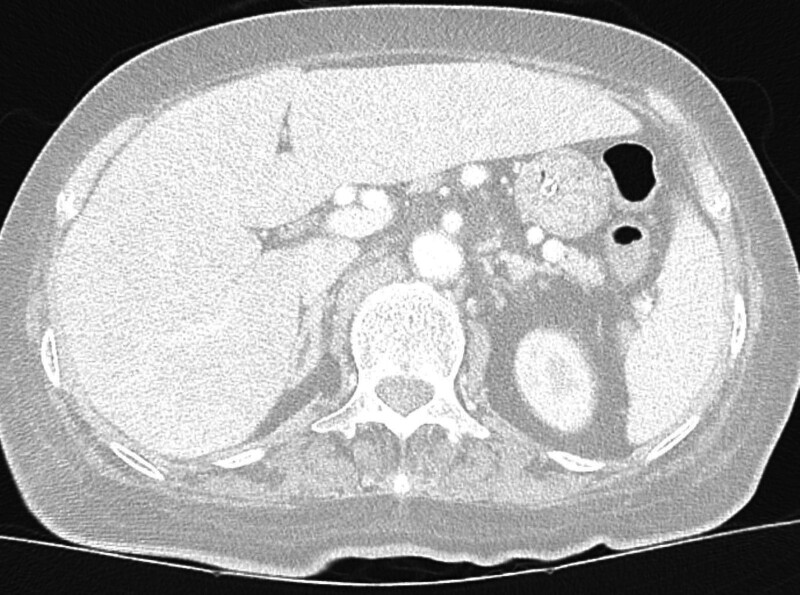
Follow-up abdominal CT scan demonstrates complete resolution of pneumoperitoneum. There is no visible free intraperitoneal air under the diaphragm or between bowel loops. The abdominal viscera, including the stomach and bowel, show normal distribution, and no signs of visceral perforation or inflammatory changes are noted. CT = computed tomography.
